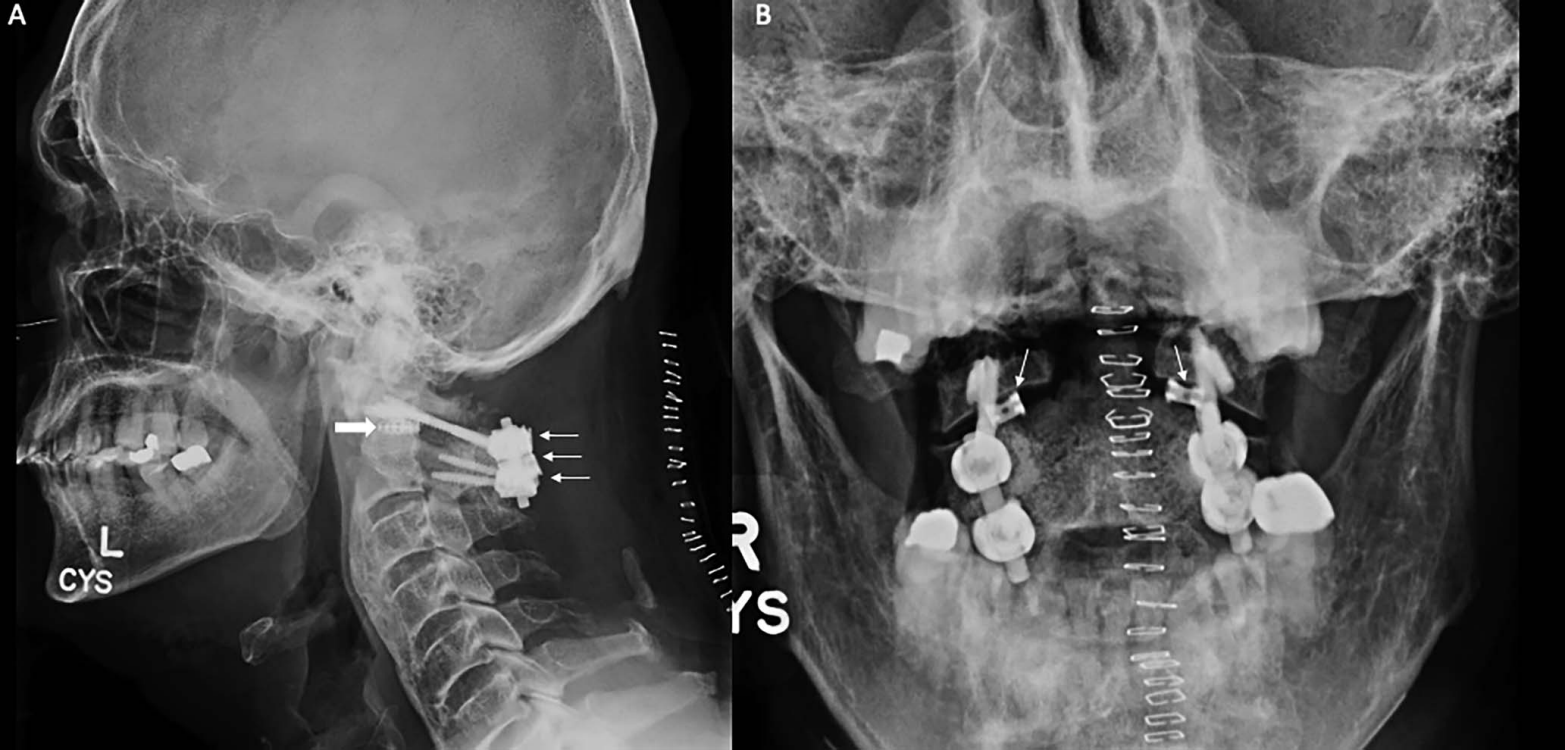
Postoperative plain films of the craniovertebral junction. (A) Lateral view shows successful placement of revision hardware across C1 and C2 with bilateral lateral mass and pars screws (arrows), along with intrafacet implants at the lateral atlantoaxial joints (block arrow). (B) Open mouth anteroposterior odontoid view shows standard posterolateral construct combined with bilateral intrafacet C1-C2 spacer placement (arrows).
